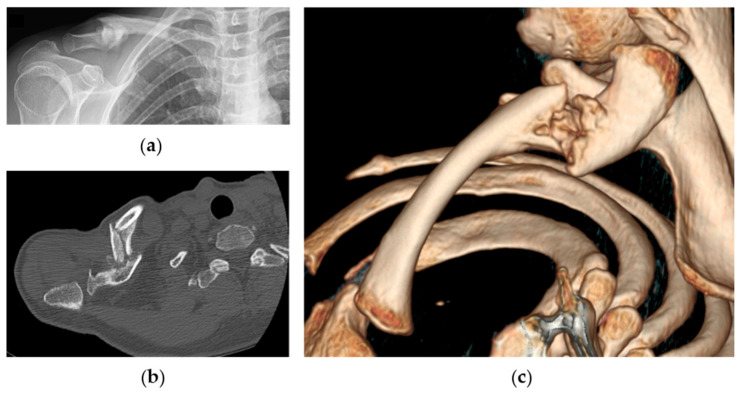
Radiographic images of the same patient showing right clavicle nonunion at 4-month follow-up: (a) anteroposterior X-ray view of the clavicle; (b) axial CT-scan evaluation of nonunion and; (c) 3D reconstruction.
